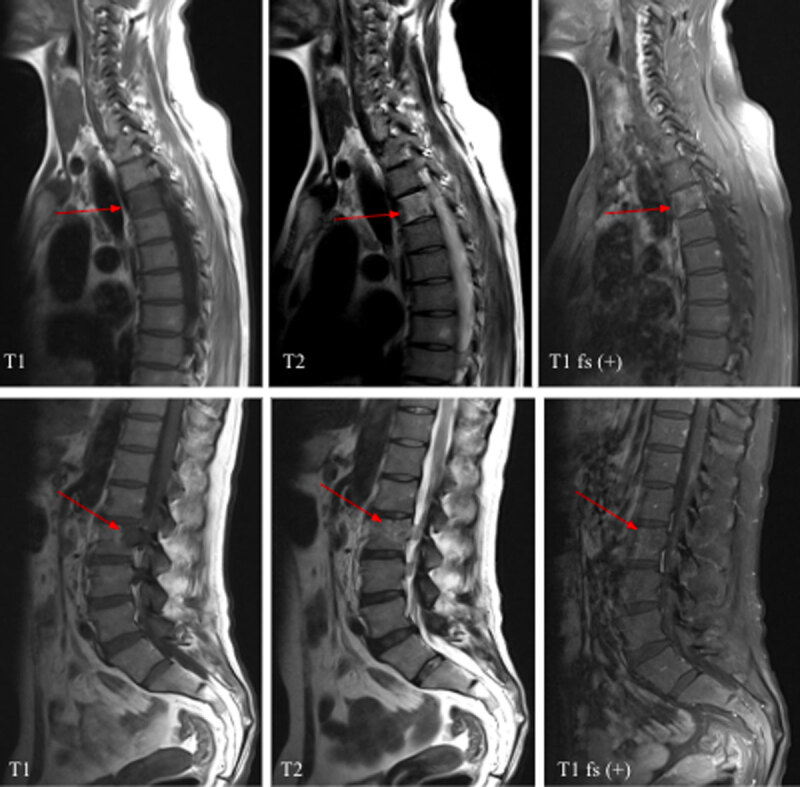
Pre- and post-contrast MRI: Enhancing lesions with hypointense on T1WI and iso/hyperintense on T2WI were noted at T3 and L2 vertebral bodies (arrows).
